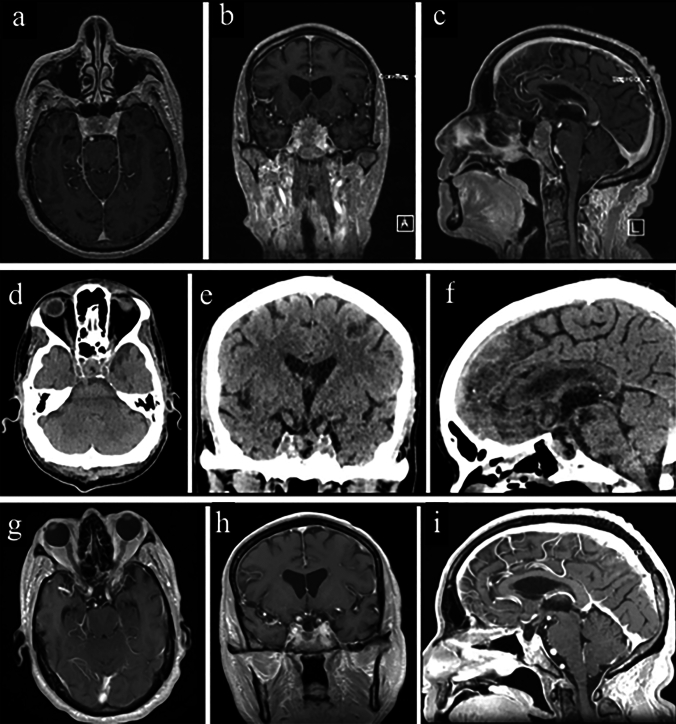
The images show radiological studies of the patient from the second case report. a, b & c Axial, Coronal, and Sagittal images from pre-operative brain MRI (T1-weighted post-contrast-enhanced images) revealed a heterogeneously enhancing sellar/suprasellar mass measuring approximately 26 × 16x19 mm. (d, e & f) Axial, Coronal, and Sagittal images from post-operative head CT show no signs of surgical site bleeding, pneumocephalus, or other complications. g, h & i Axial, Coronal, and Sagittal images from postoperative brain MRI (T1-weighted post-contrast-enhanced images) showing a gross total resection of the tumor
